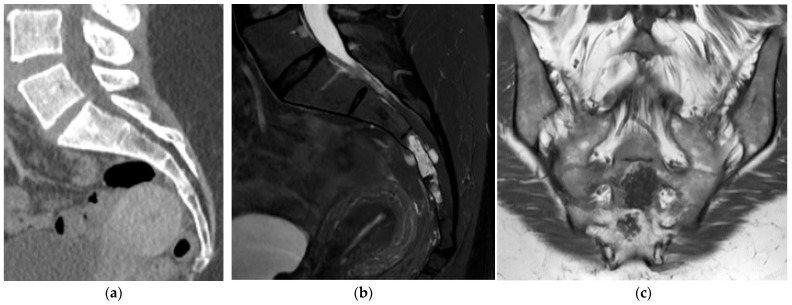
Benign Notochordal Cell Tumors. (a) Sagittal CT, very mildly increased sclerosis in the S3 body. (b) Sagittal STIR MR, hyperintense lesion in S3 without cortical expansion or soft tissue component. A second lesion of similar imaging characteristic is present in S4. The small cystic lesion in the adjacent sacral canal represents a perineural cyst. (c) Coronal oblique T1 MR: lesion demonstrates low T1 signal.
